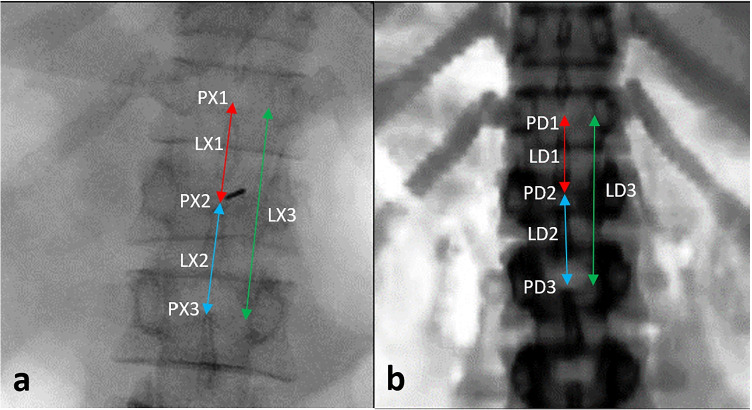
The source to detector direction localization: Intervertebral distance measurement between different vertebral levels in X-ray image (a) and DRR image—generated after initial spatial localization (b). Source: Orthopaedics and Radiology Department - KMC Manipal
